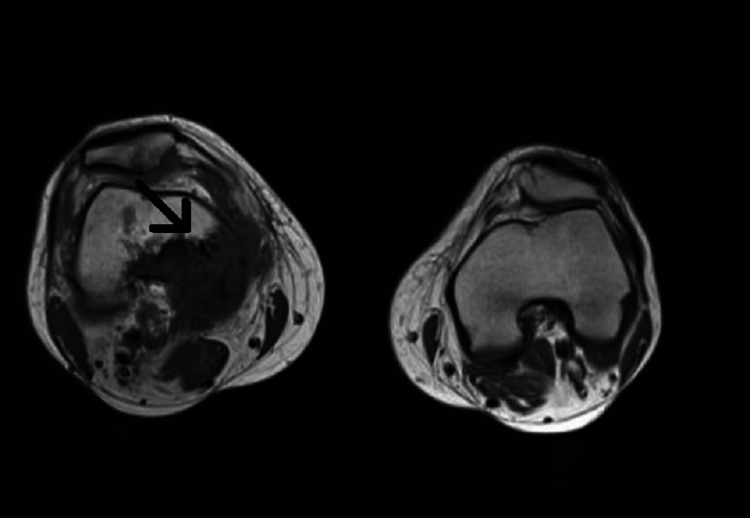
MRI of the knees Mass with cortical disruption, cystic areas, sclerosis, and adjacent soft tissue involvement
